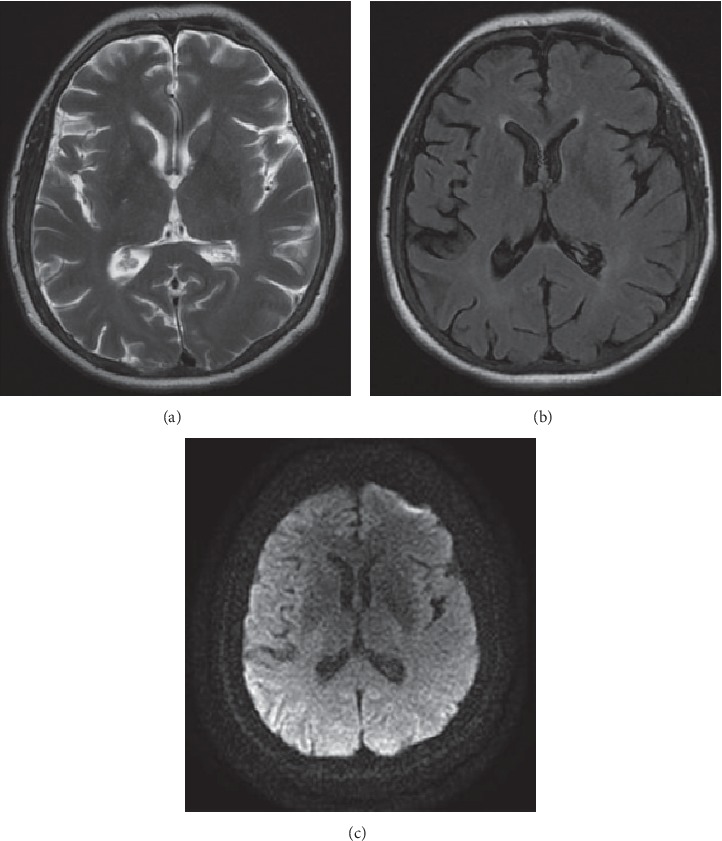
Follow-up brain MR-T2 (a), FLAIR (b), and DWI (c) axial images obtained in another facility 1 month after the initial MR shows complete resolution of the lesions.
